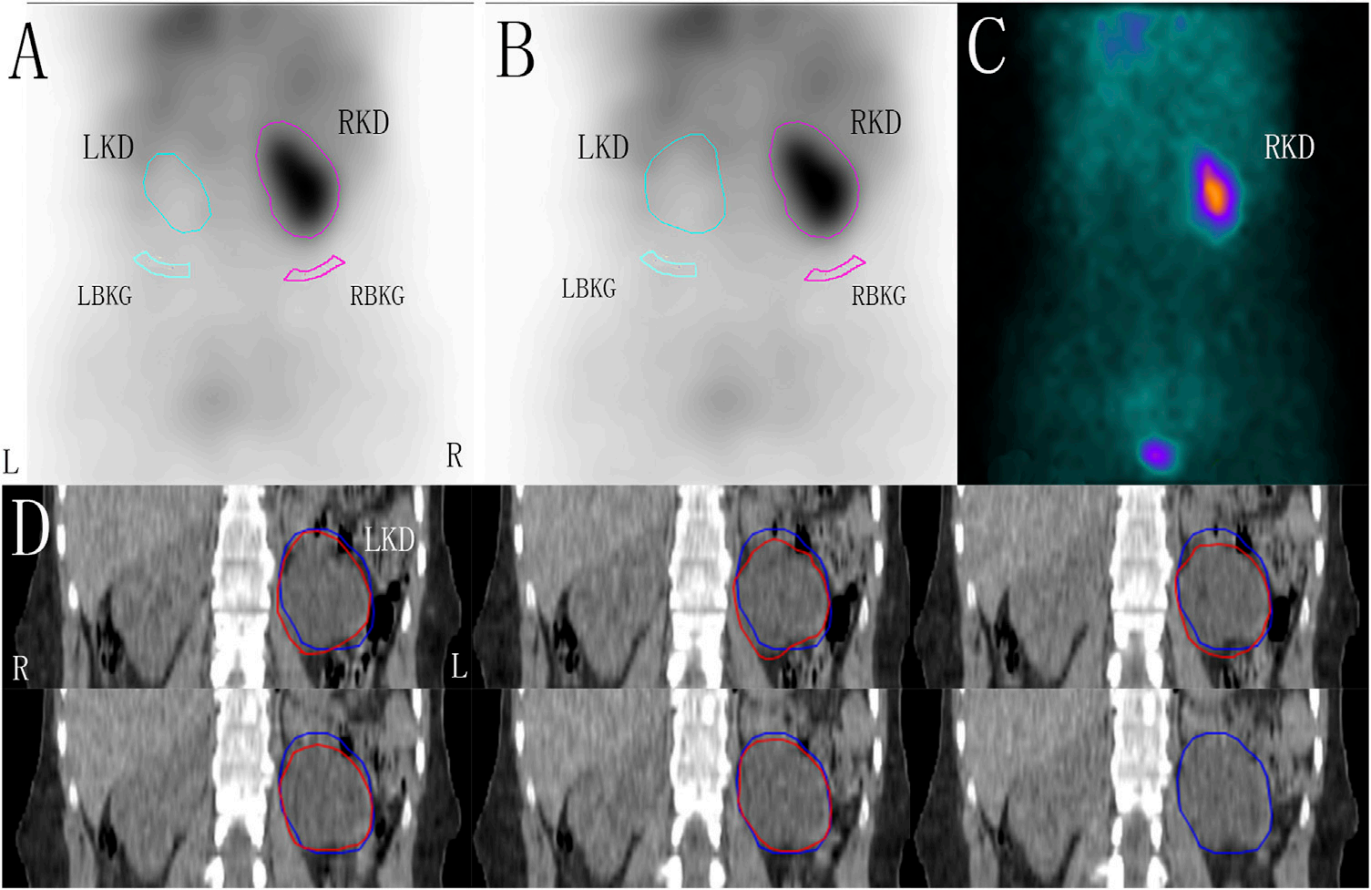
The renal region of interest (ROI) was corrected by computer tomography (CT) in a patient with left hydronephrosis, zoom: 1.5. (A) ROI of the kidneys and the background drawn using the Gates method. (B) Based on the maximum renal ROI area corrected by CT, the ROI of the kidneys and the backgrounds was drawn using the Gates method. (C) One-minute frames: shows the frame-per-minute image overlaid by the kidneys, cortex, background, and bladder. (D) ROIs of the left kidney were delineated layer by layer on CT coronal sectional images (red line), and the maximum ROI area of the kidney were redrawn and used as the renal ROI area from CT (blue line). LKD, left kidney; RKD, right kidney; LBKG, left background; RBKG, right background.
